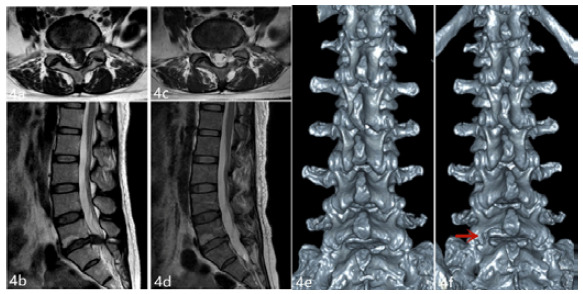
Representative lumber disc herniation case pre- and post-operation (L5-S1, left paracentral type, female). Preoperative lumbar MRI showed that the L5-S1 intervertebral disc was herniated to the left and that the S1 nerve root and dural sac were compressed (a, b); 12 months after the operation, lumbar MRI showed that the herniated intervertebral disc tissue had disappeared and that the left S1 nerve root and dural sac compression was relieved (c, d); Comparison of a three-dimensional CT reconstruction of the lumbar spine before the operation and 12 months after it (the arrow indicates the increase of the L5-S1 left interlaminar bone window after the operation) (e, f).
